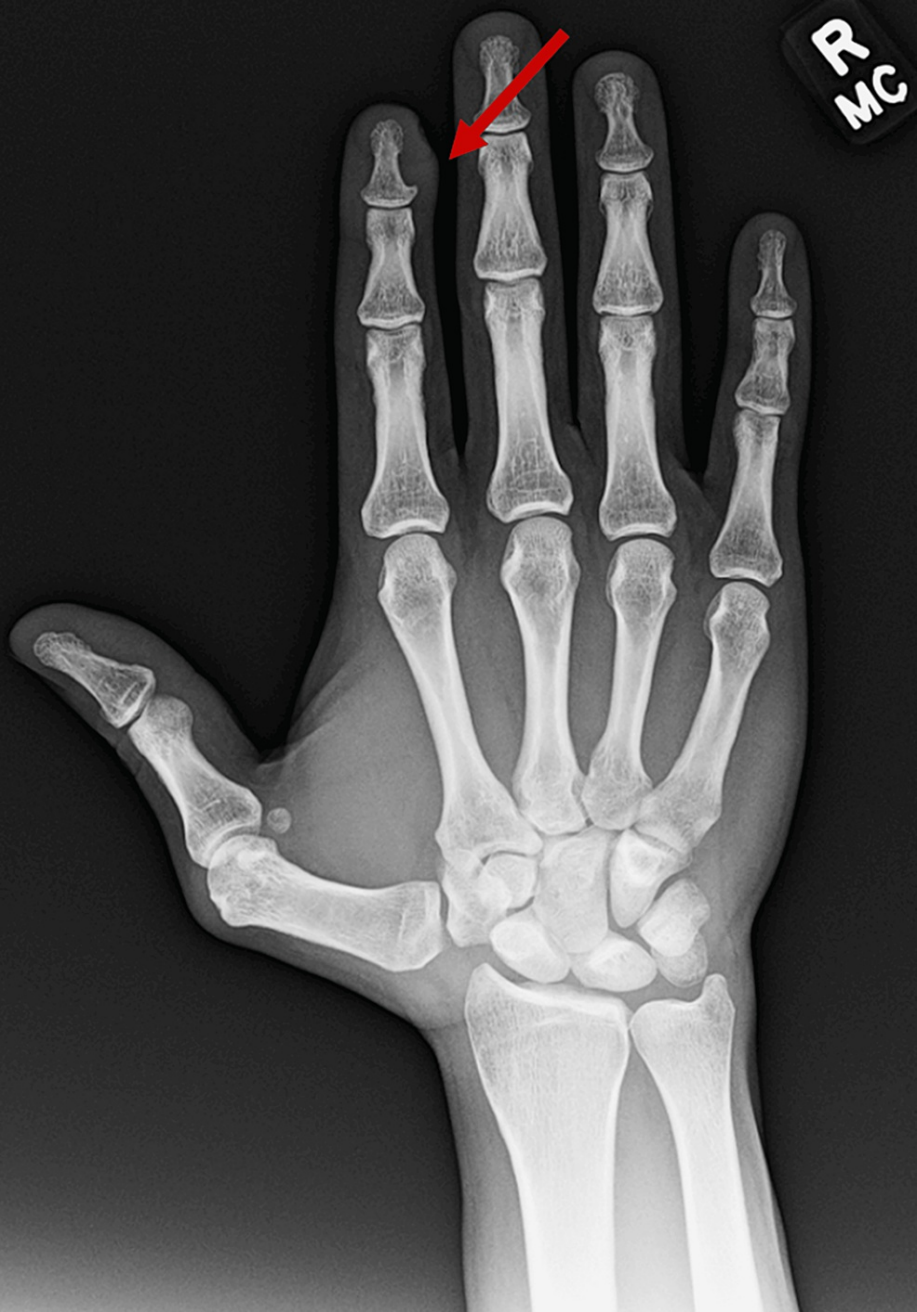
Plain radiograph of the right hand.
